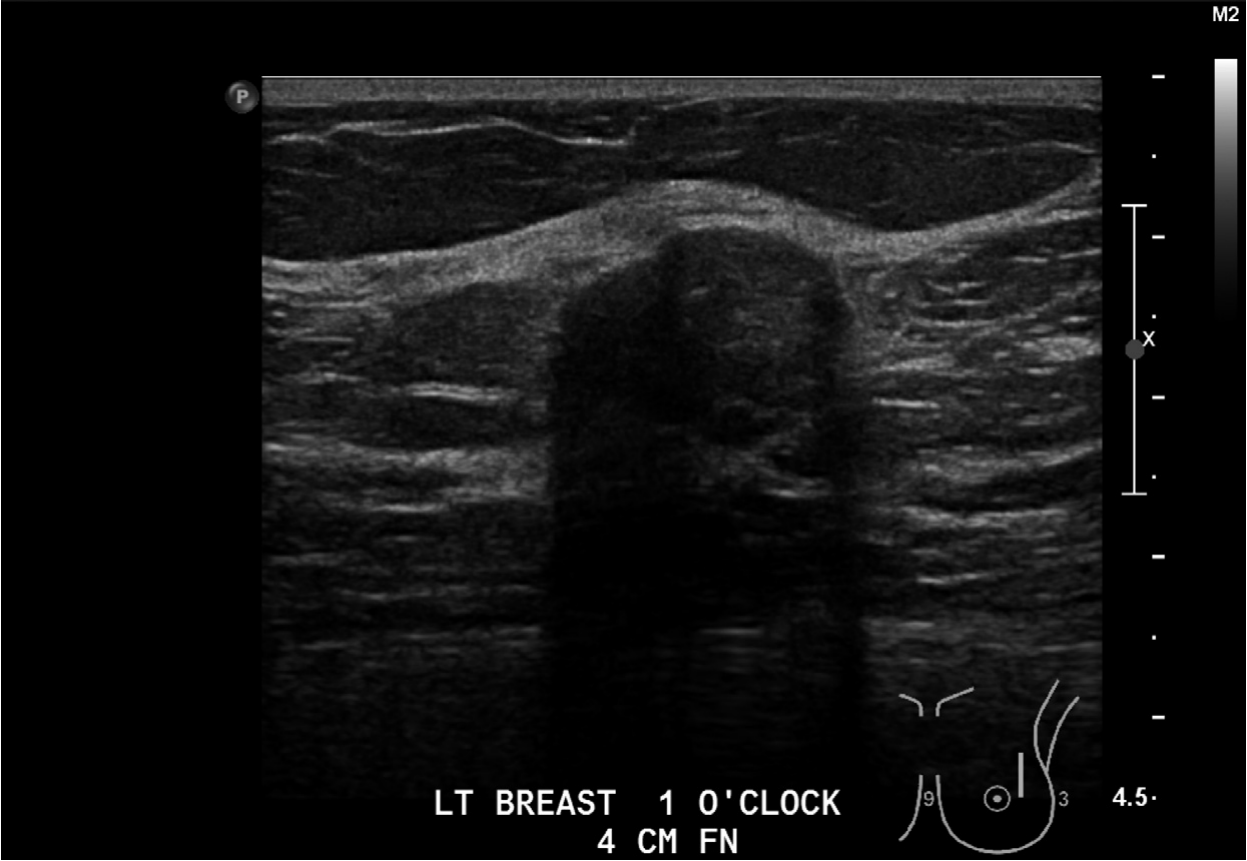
Ultrasound image demonstrating a fibroadenoma. Image courtesy of BreastScreen ACT.
